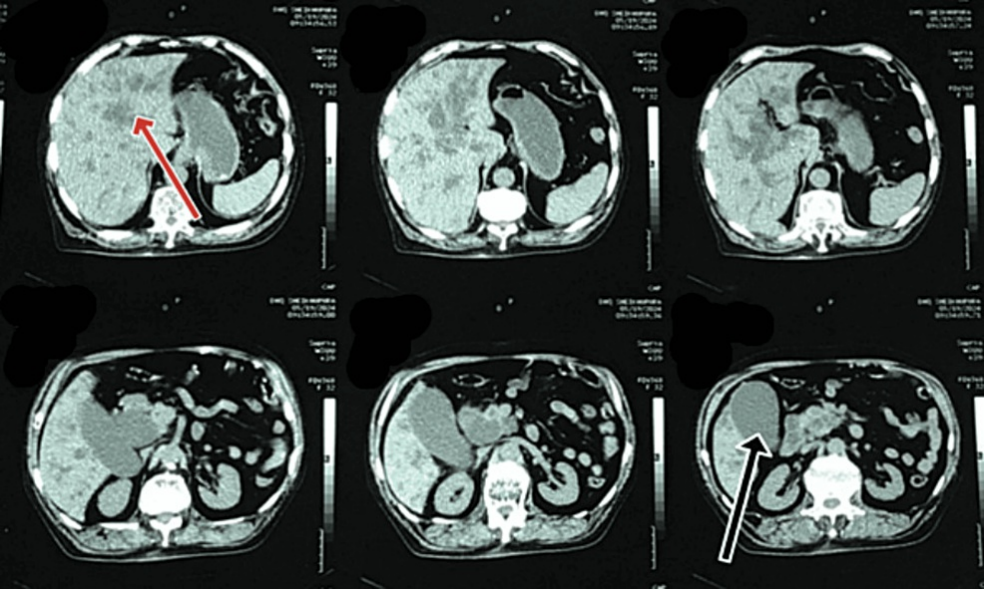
CT of the abdomen and pelvis without contrast showing moderate dilation of the CBD lumen proximal to IHBCs and significant distension of the GB lumen The red arrow shows the IHBC dilatation. The black arrow shows the distended GB. CT, computed tomography; CBD, common bile duct; IHBC, intrahepatic biliary channel; GB, gallbladder
